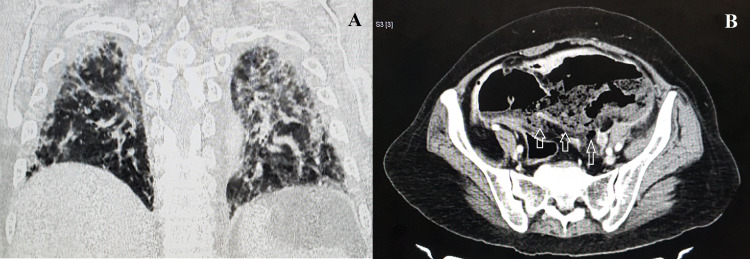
CT of the chest showing centrilobular ground glass opacities involving both the lungs and suggestive of COVID-19 pneumonia (A) and grossly dilated sigmoid colon with imperceptible posterior wall (arrows) (B). CT: computed tomography; COVID-19: coronavirus disease 2019
